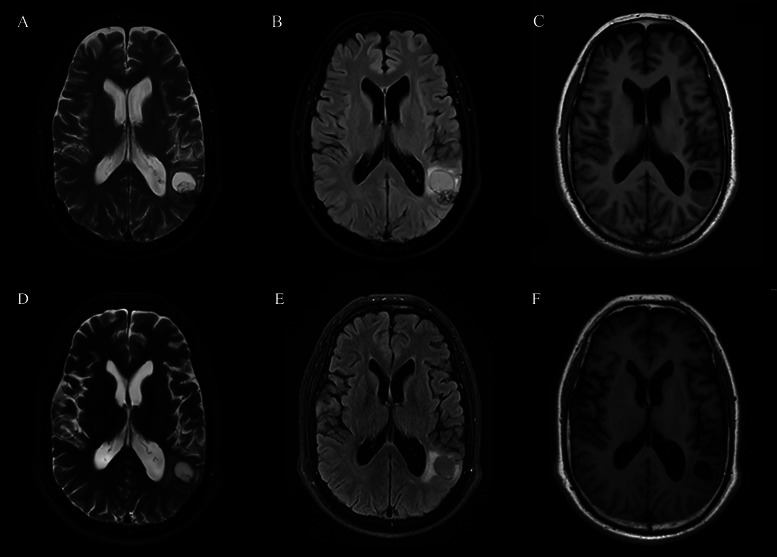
MRI shows that the largest cystic nodule was located next to the left ventricular triangle: (A–C) half a month before antiparasitic treatment and (D–F) half a month after antiparasitic treatment.
